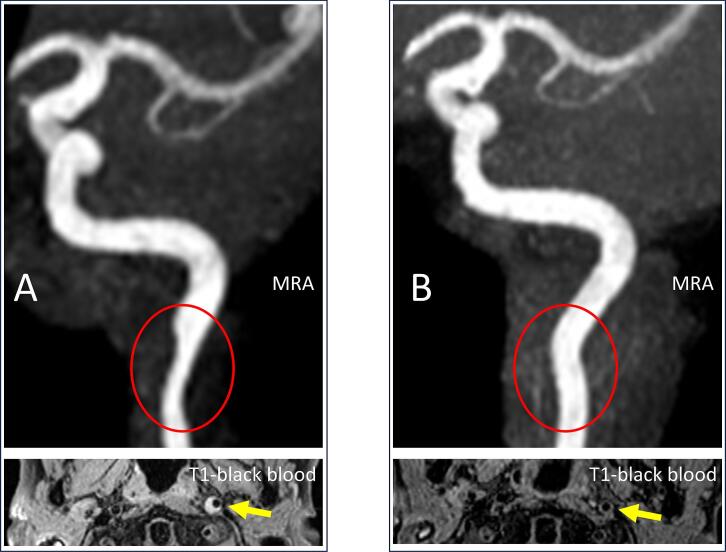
Follow-up magnetic resonance (MR) imaging (MRI). Stenosis on MR angiography and intramural hematoma on T1-weighted black blood imaging (yellow arrow) (A) shows alleviation on follow-up imaging 6 months after onset (B). (For interpretation of the references to colour in this figure legend, the reader is referred to the web version of this article.)
